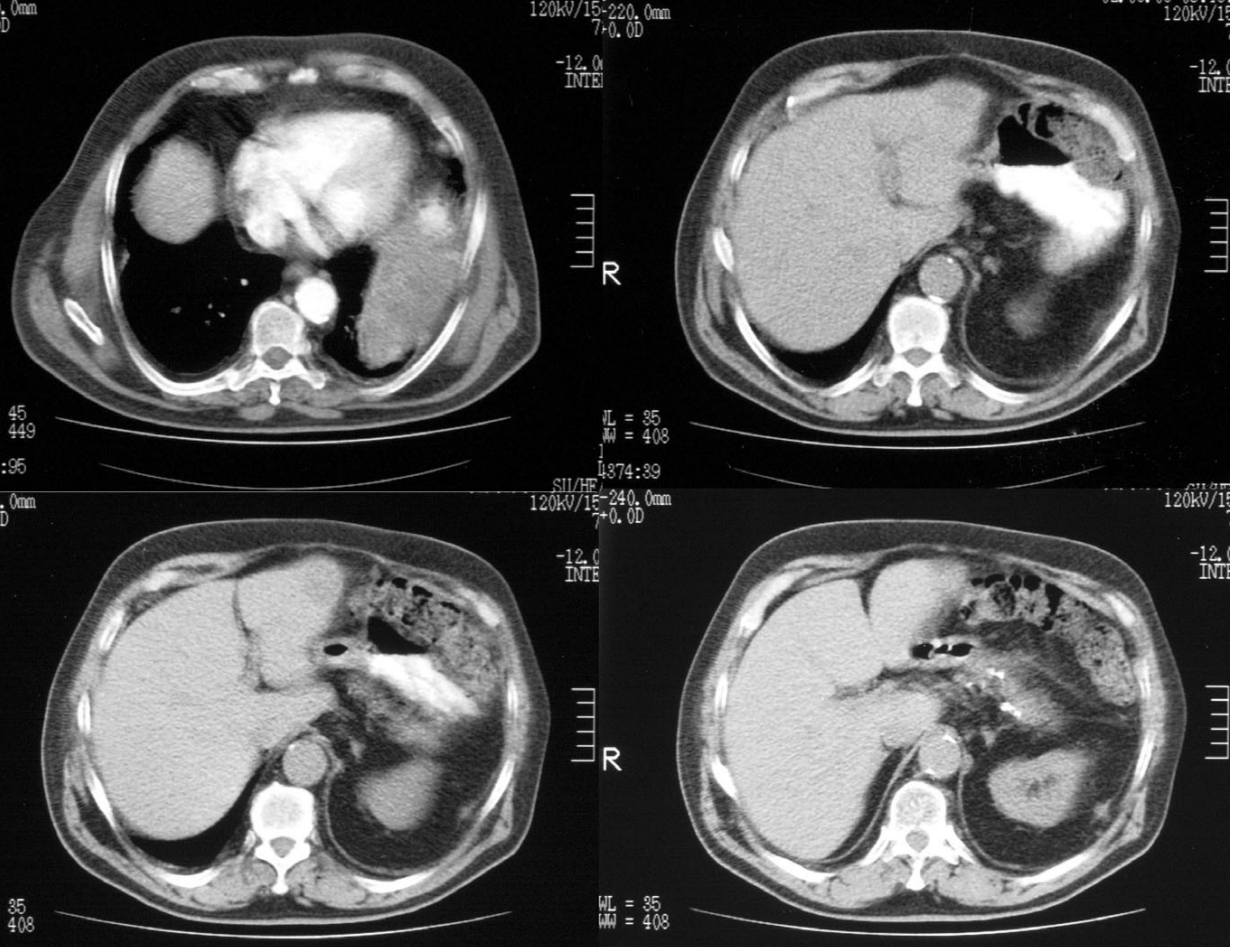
CT scan after the treatment.
